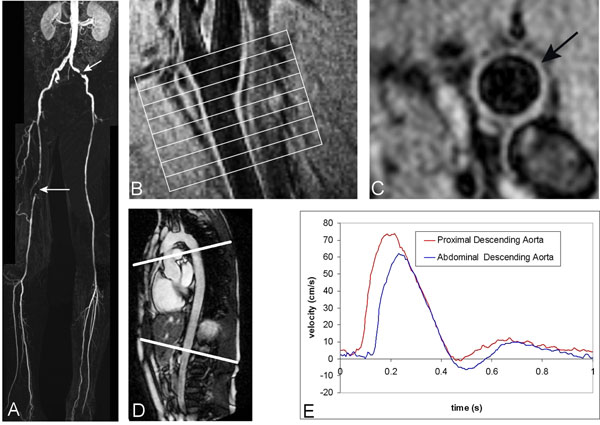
(A) Coronal contrast-enhanced MR angiographic maximum intensity projection images of 67-year-old man presenting with bilateral claudication and significant stenosis in the left external iliac artery (short arrow) and an occlusion in the right superficial femoral artery (long arrow); (B) Sagittal black-blood image of the carotid bifurcation presenting positioning of carotid vessel wall sampling in the common carotid artery (C); (D) Sagittal image of the aorta presenting positioning of two one-directional through-plane velocity-encoded MRI acquisitions at the proximal and abdominal descending aorta. Pulse Wave Velocity is determined from wave propagation analysis between measurement sites (E).
